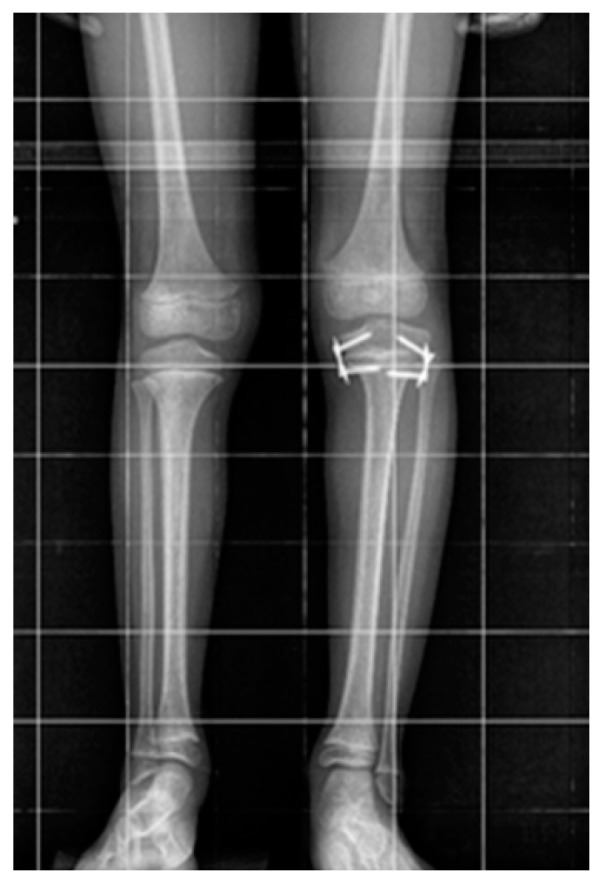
Postural X-ray of a patient treated for LLD with eight-plate implants.
